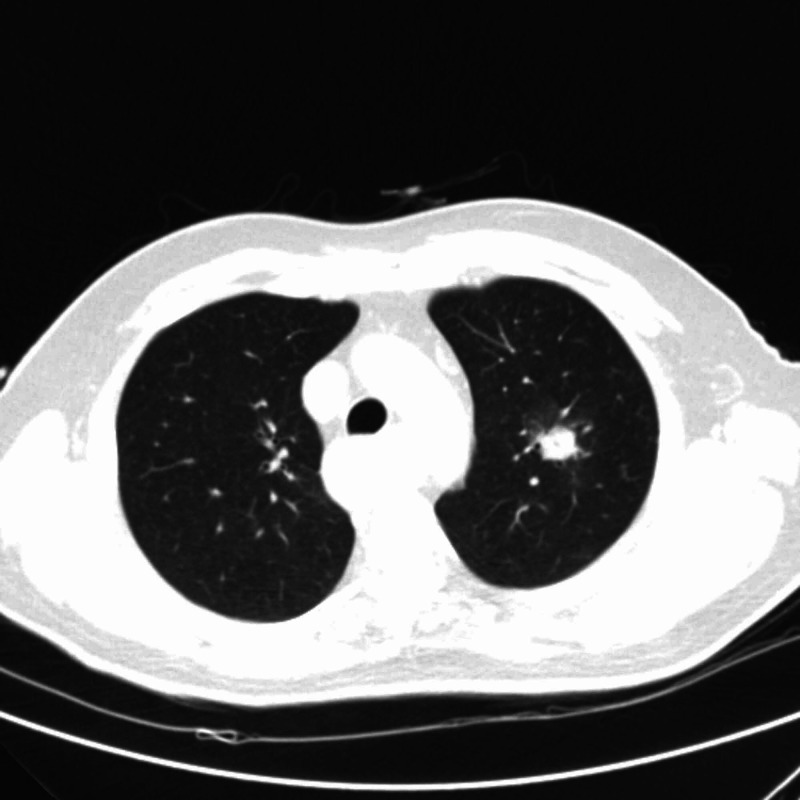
Chest CT scan Presence of a spiky, solid image in the upper left lobe, with 22 mm diameter and ground-glass opacity, highly suggestive of a neoplastic process. CT: computed tomography
